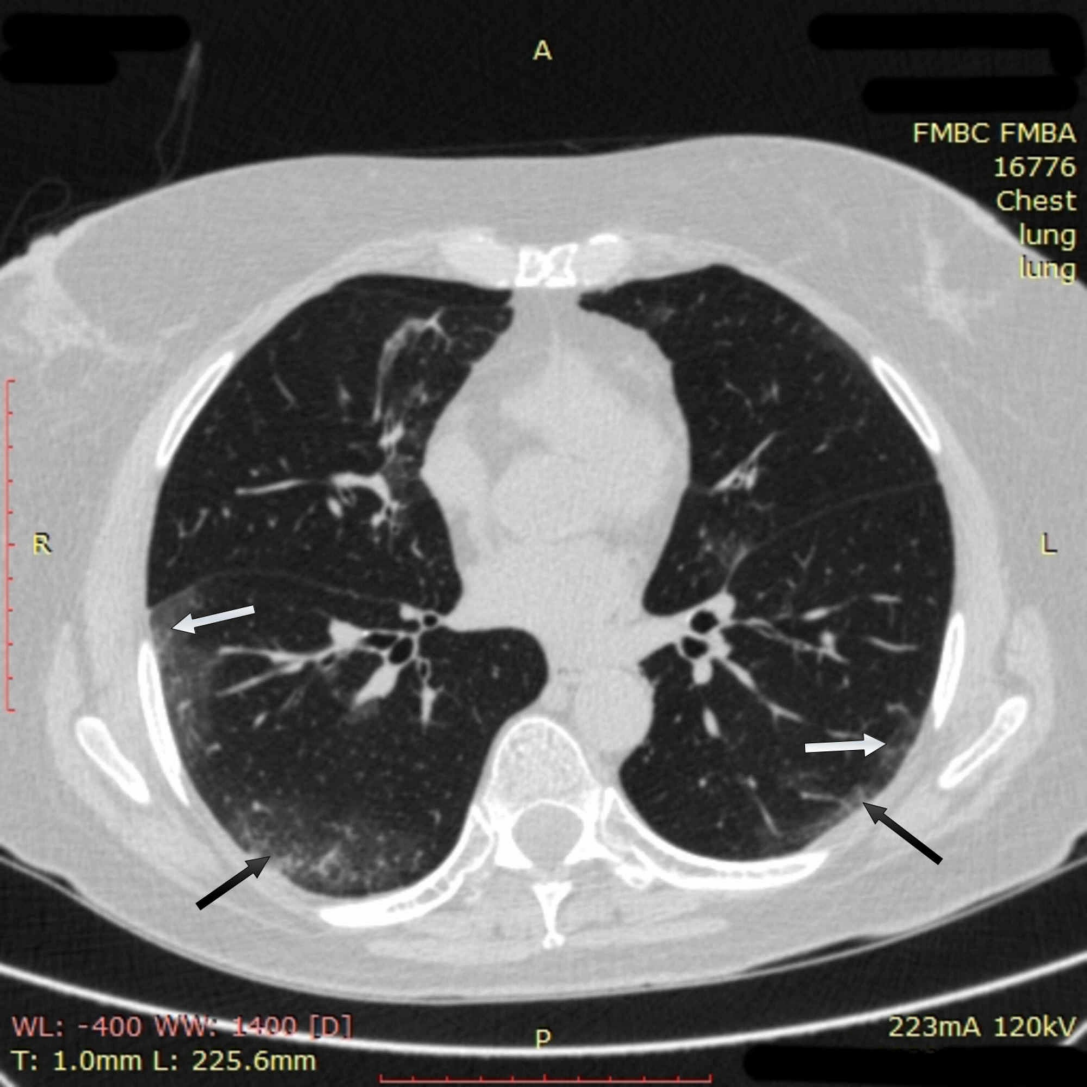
Chest CT imaging of patient 1 on admission CT imaging on admission showed multiple bilateral ground-glass opacities (white arrows) in subpleural areas of lower and middle regions of both lungs; reticular changes in the same zones were also observed (black arrows) CT: computed tomography
